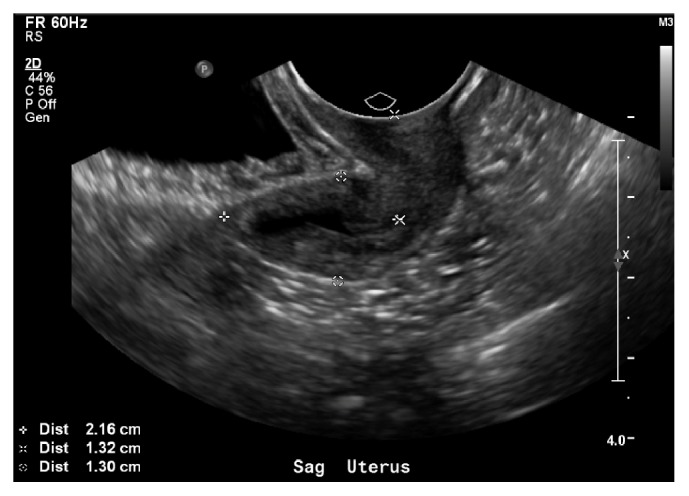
Transvaginal ultrasound of uterus.
